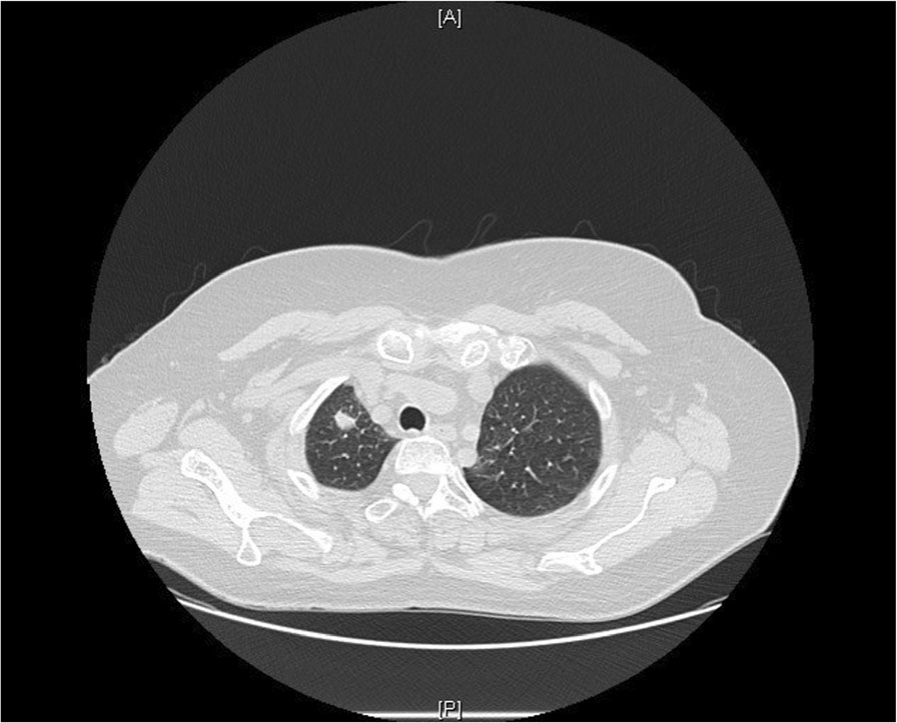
CT scan showing pulmonary right upper lobe nodule
